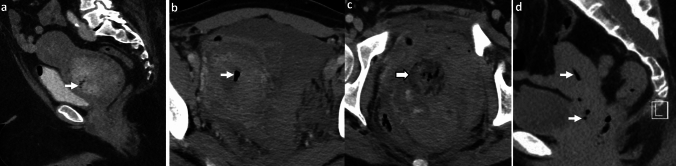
Pitfalls for gas gangrene of the uterus. a Post-embolization changes. CT image shows expected air foci within cervical fibroid post-uterine artery embolization (solid white arrow, a). b, c Post-partum and surgical changes. Patient with full-term vaginal delivery complicated by vaginal hematoma, post incision-drainage and hemostatic packing. CT images show expected post-partum air in the uterine cavity (solid white arrow, b) and para-uterine lucencies secondary to hemostatic pack (block white arrow, c). d Post-procedure changes. Air in the endometrial cavity and endocervical canal (solid white arrows, d) after removal of the intrauterine device
